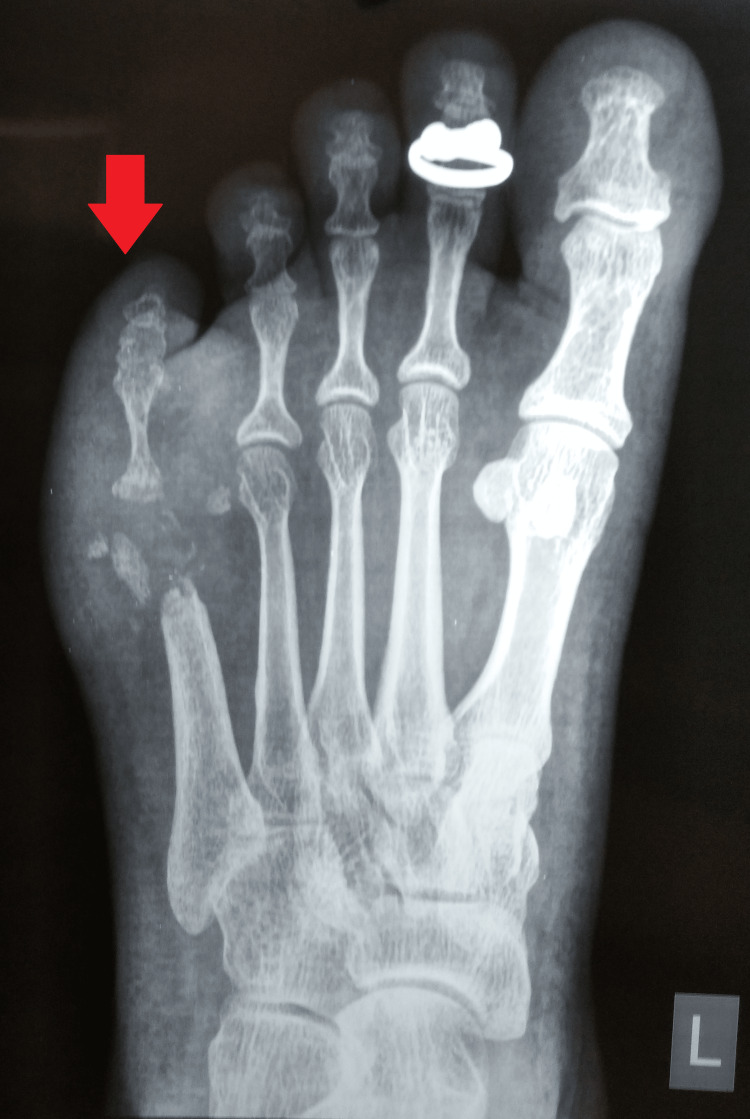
Antero-posterior radiograph of the left foot
